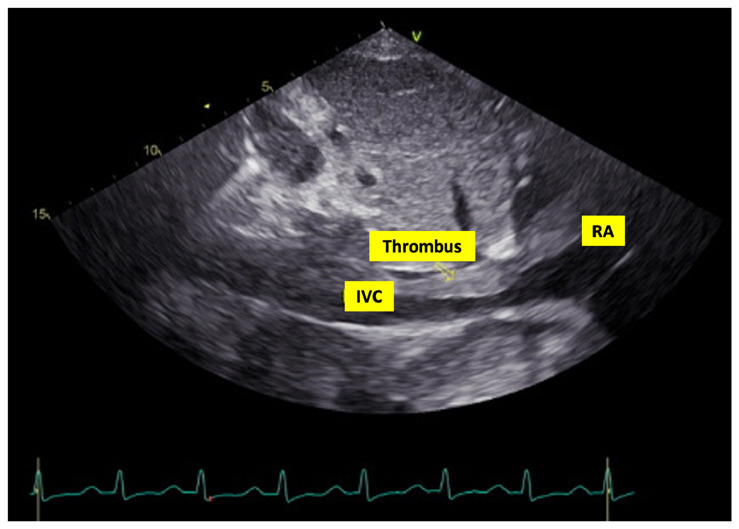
Transthoracic echocardiogram (TTE) showing thrombus in the inferior vena cava. RA = right atrium; IVC = inferior vena cava.
